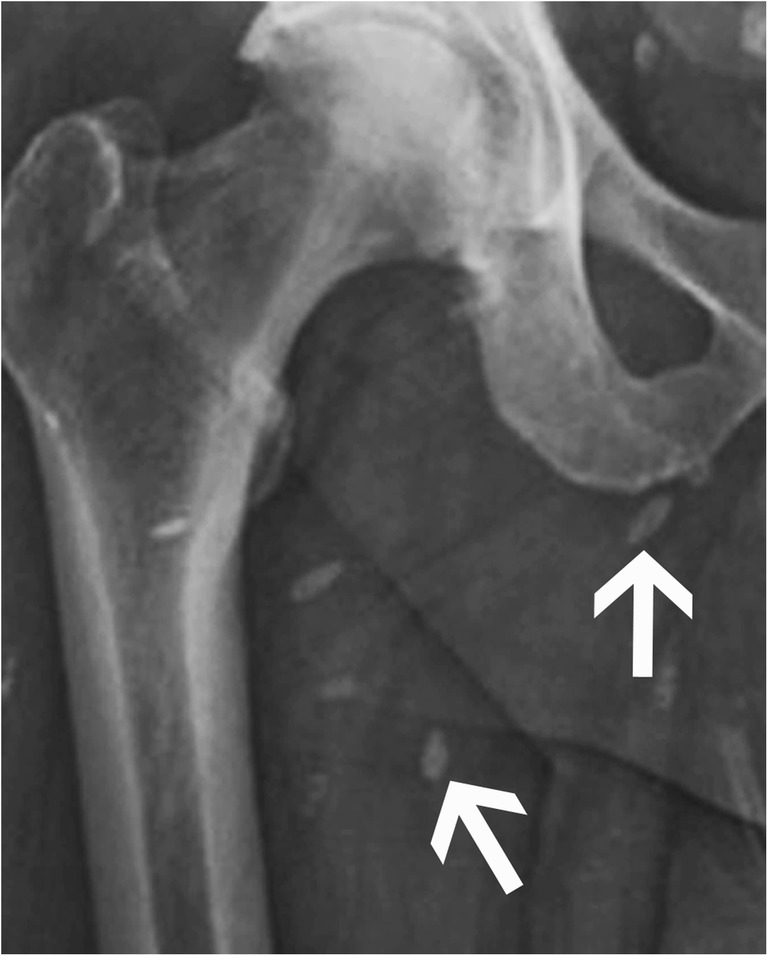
Cysticercosis. Anteroposterior radiograph of the pelvis shows multiple cigar-shaped calcifications characteristic of cysticercosis
